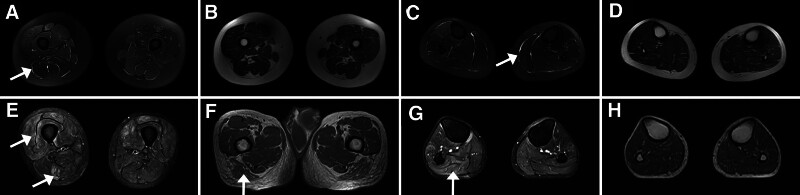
MRI changes in anti-MDA5-positive JIIMs. (A–D) Hyperintense STIR signal in fascia (white arrows) with normal T1 signal of thighs and lower legs in Patient 2. (E) Hyperintense STIR signal (white arrows) in anterior and posterior part of thighs in Patient 3. (F) Hyperintense T1 signal (white arrow) in posterior part of thighs in Patient 3. (G and H) Hyperintense STIR signal (white arrow) without hyperintense T1 signal of posterior lower legs in Patient 3. JIIM = juvenile idiopathic inflammatory myopathy; MDA5 = melanoma differentiation associated gene 5; MRI = magnetic resonance imaging; STIR = short-tau inversion recovery.
